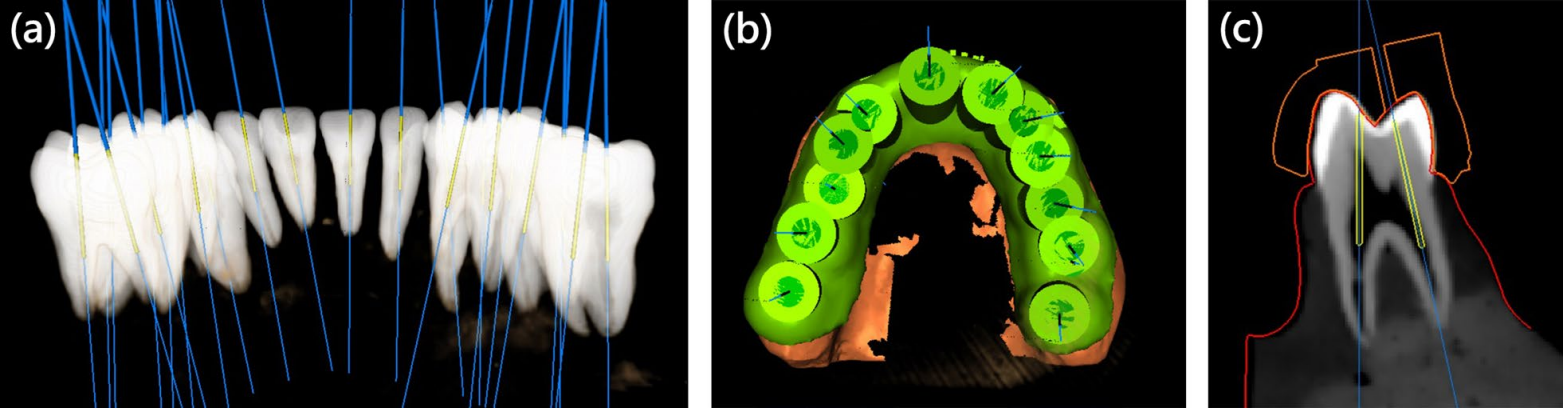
Virtual planning in implant software: a Virtual access planning: 3D image reconstruction of cone beam computed tomography and virtual burs with axis within the range of conservative endodontic access cavity. b Template with resin sleeve designed in software. c Virtual planning in premolar. Template (orange outline), scan surface (red outline), virtual bur (yellow cylinder), and cone beam computed tomography were superimposed in software
